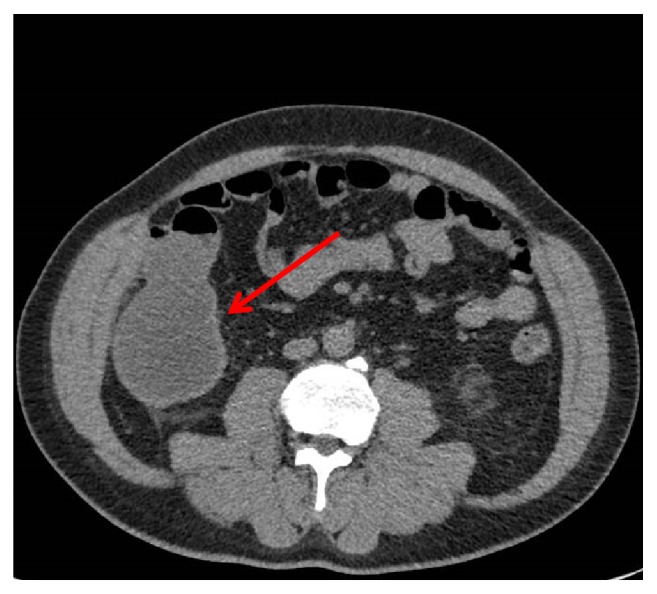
CT KUB showing the cystic swelling arising from the appendiceal stump (red arrow).
